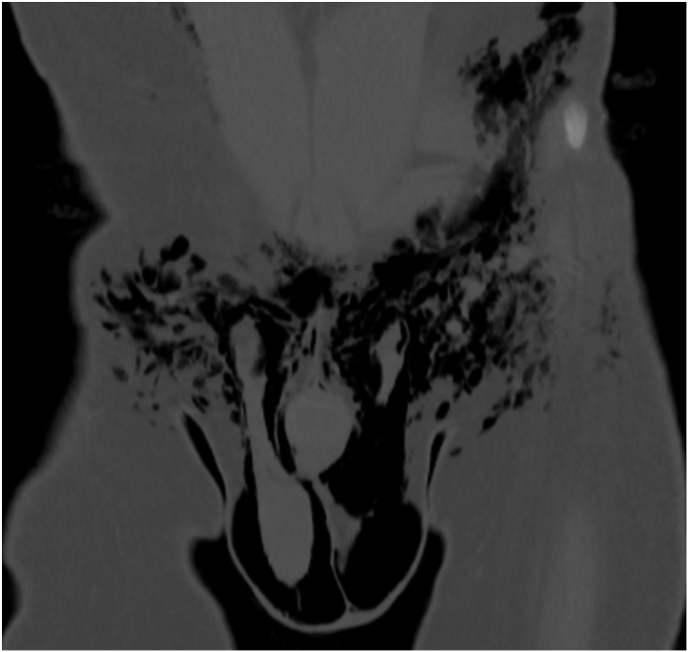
Non-contrast-enhanced computed tomography (CT) scan of the abdomen and pelvis demonstrating extensive subcutaneous emphysema localized to the superficial fascial planes of the scrotum and lower anterior abdominal wall.
